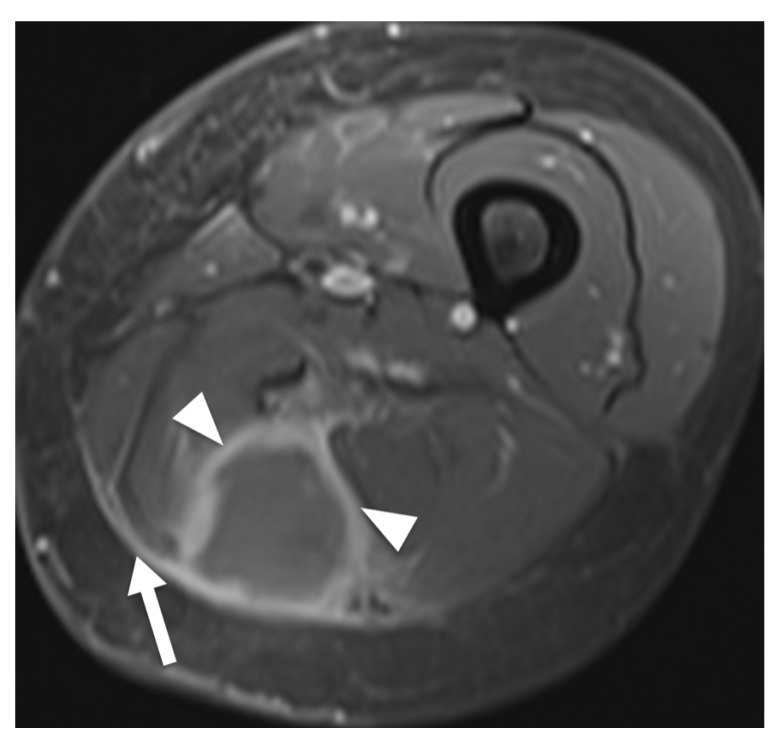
MRI of a 68-year-old woman presenting with non-Hodgkin lymphoma manifestation in the thigh. Transversal contrast enhanced fat-saturated T1-weighted image shows a tumor in the posterior compartment of the thigh, revealing predominant enhancement of tumor margins (arrowheads). Moreover, signal alteration of fascia lata can be noted (arrow).
